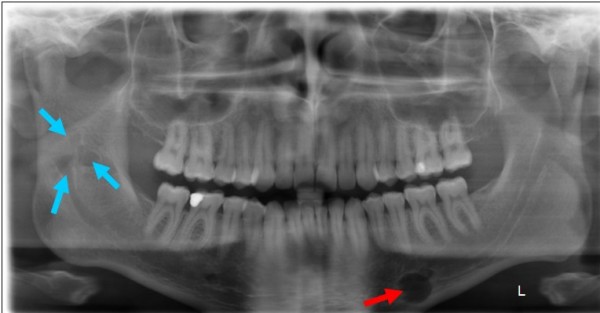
Panoramic radiography (patient, 23 years): 13 mm osteolysis in left mandible (red arrow). Noted translucency within the right ramus (blue arrow).
